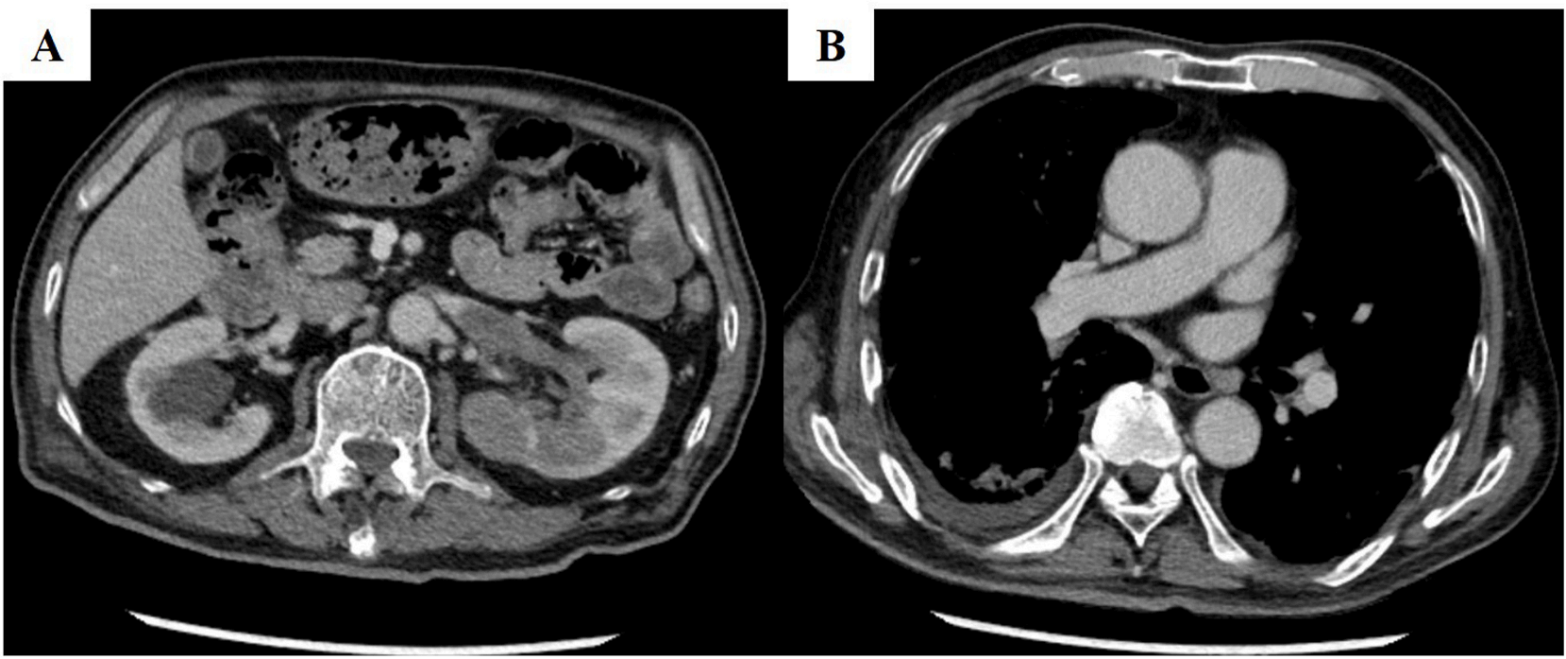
Images of enhanced-contrast CT findings at diagnosis of renal cell carcinoma. (A) An internal heterogeneous mass in the morphologically preserved left kidney and invasion of the left renal vein are shown. (B) There were no massive tumor emboli in large pulmonary arteries.
